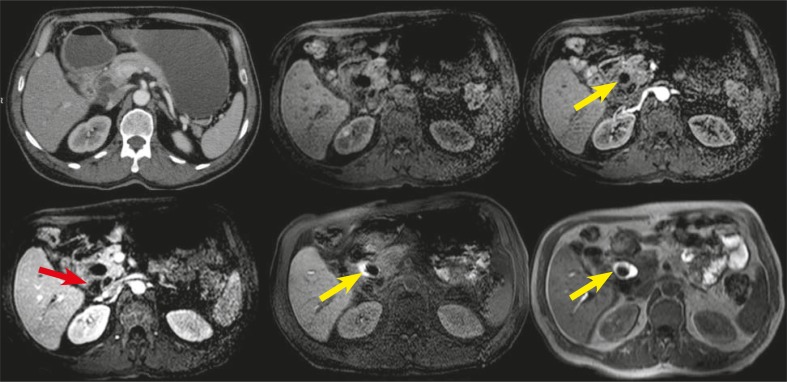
Axial contrast enhanced multidetector computed tomography (upper left), noncontrast-enhanced T1 weighted (upper medium), arterial phase contrast-enhanced T1 weighted (upper right), portal phase contrast-enhanced T1 weighted (left below), delayed phase contrast-enhanced T1 weighted with and without fat saturation (middle below and right below, respectively) images of the patient. Pancreatic cystic lesion with contrast-enhanced thin septi-wall (red arrows) can be differentiated from ductus choledochus (yellow arrows) by gadoxetic-acid enhanced T1 weighted images. Also, delayed phase T1 weighted images show stone in the ductus choledochus.
